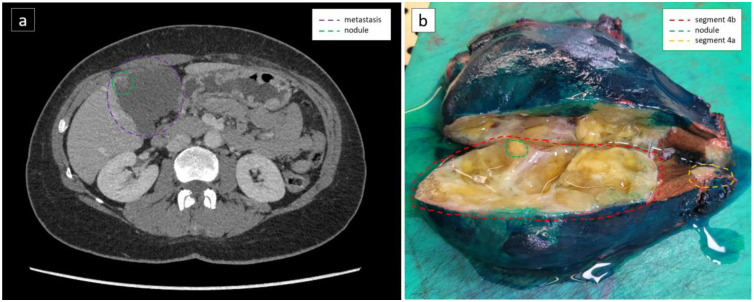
Liver metastasis on CT scan and after resection: (a) CT scan two months prior to surgery. The purple encircled part represents the complete liver metastasis. The green encircled part represents the regenerative nodule in a mass; (b) Cut-open resection of liver segments 4a and 4b, encircled in yellow and red, respectively. Segment 4b contains the regenerative liver ‘nodule within the mass’, encircled in green, directly after surgery.
